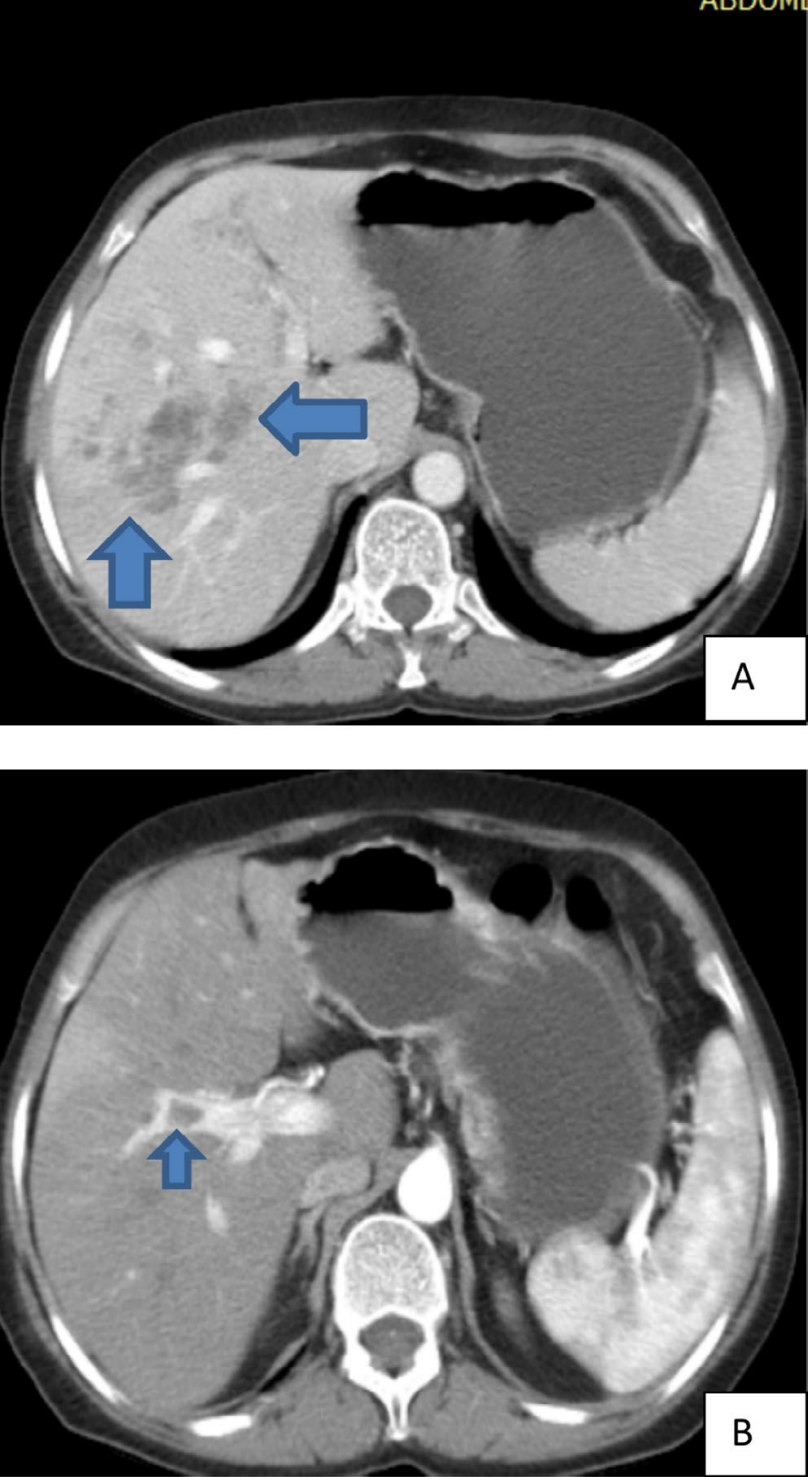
(A, B) Contrast‐enhanced abdominal computed tomography: (A) multiple hypo‐enhancing focal hepatic lesions (arrows) noted within the liver parenchyma, most appearing along the portal tracts; (B) Focal filling defect noted within the right anterior segment of portal vein (arrow).
